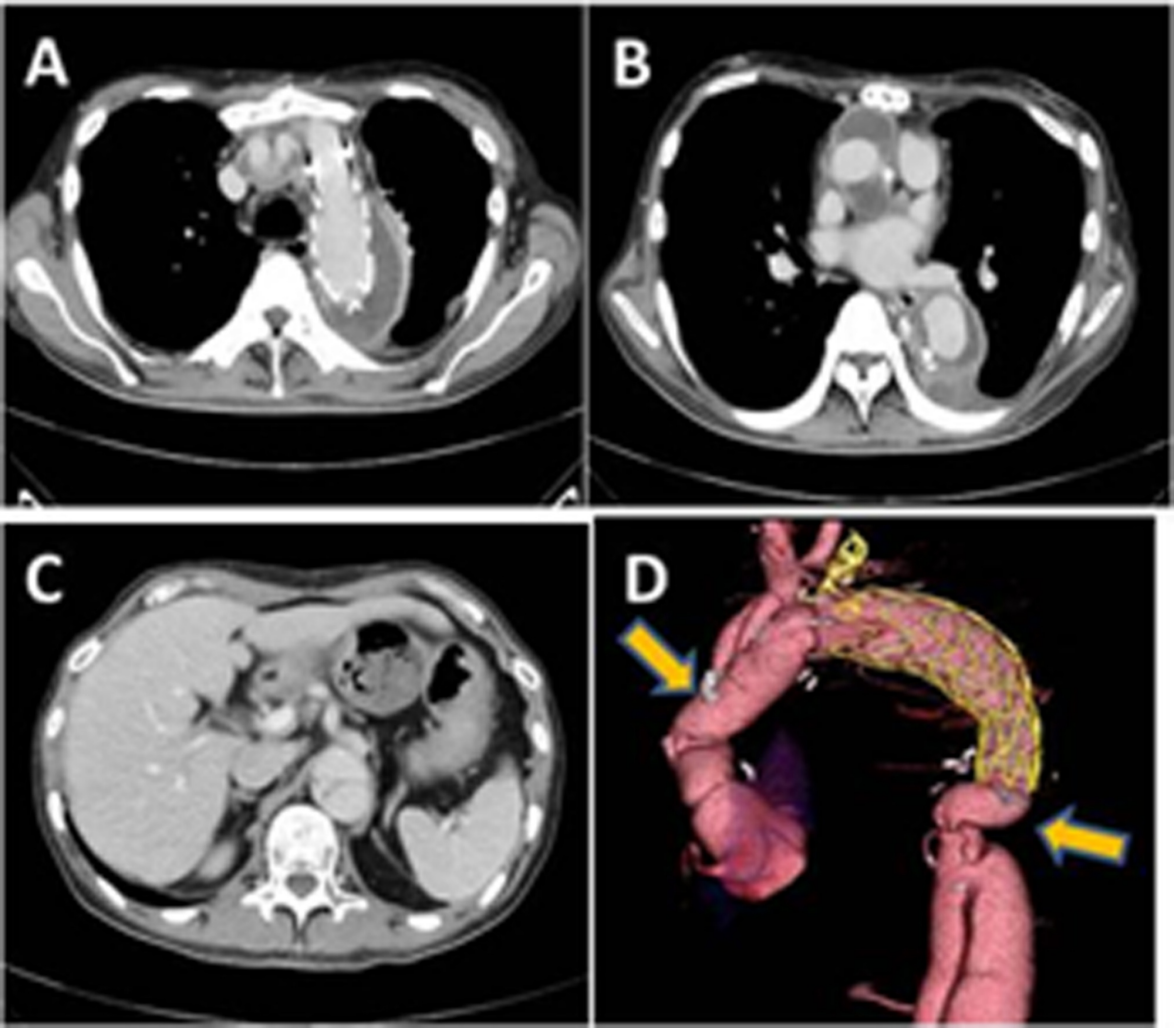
A-D. Chest computed tomography image obtained six months after the operation. No abnormal findings were observed in the replaced ascending and descending aorta (arrow). The false lumen of the descending aorta was completely thrombosed and shrunken.
